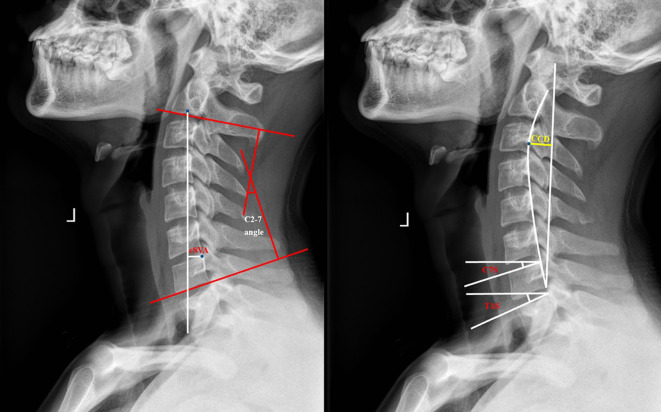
Lateral radiograph for measuring radiographic parameters. cSVA, C2-7 sagittal vertical axis; CCD, cervical curve depth; C7S, C7 Slope; T1S, T1 Slope.
